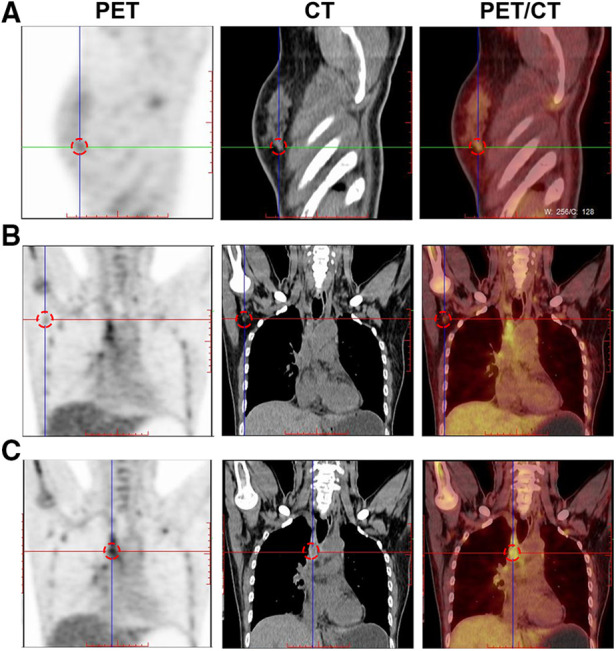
Breast molecular imaging findings from patients with invasive ductal carcinoma with micropapillary features (A) A soft, irregular tissue nodule is seen in the lower outer quadrant of the right breast, approximately 1.0 cm × 0.8 cm in size, exhibiting abnormal increased uptake of ^18^F-FDG. (B) Several small lymph nodes are visible in the right axilla. The larger ones are approximately 1.2 cm × 0.7 cm in size and exhibit mildly increased uptake of ^18^F-FDG. Abbreviations: PET, positron emission tomography; CT, computed tomography; ^18^F-FDG, ^18^F-fluorodeoxyglucose radiotracer.
